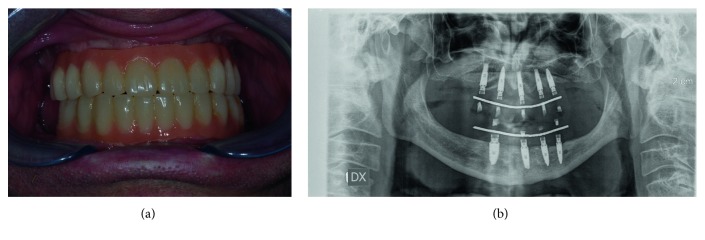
(a) Clinical and (b) radiographical aspect of the definitive restoration after 1 year follow-up.
